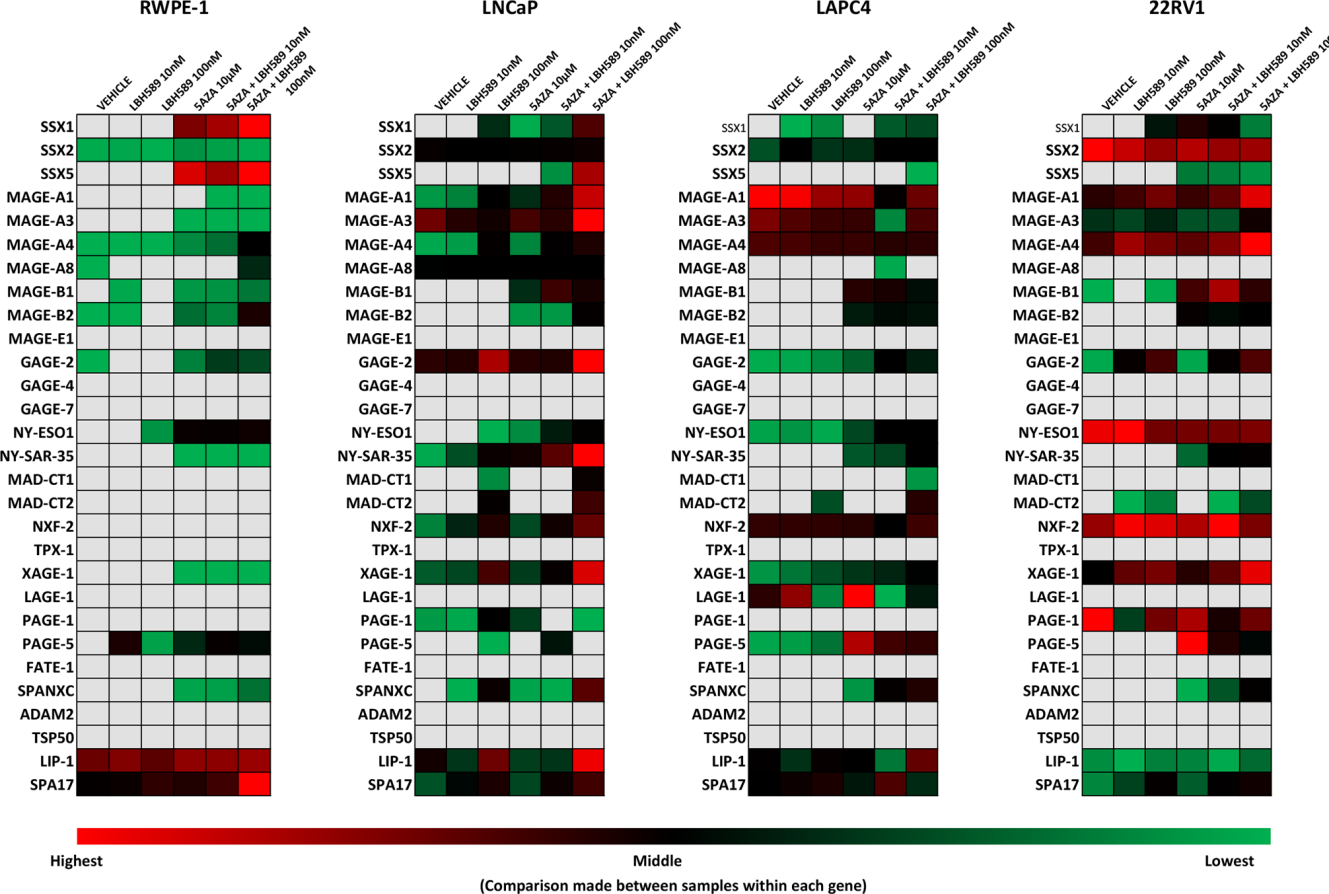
Quantitative analysis of relative expression of CTA mRNA in androgen-receptor expressing PC cell lines and in RWPE-1 normal epithelial cells in response to EMA treatment RNA was evaluated by qRT-PCR for expression relative to an internal control transcript (P0) following EMA treatment of RWPE-1, LNCaP, LAPC4, and 22rv1 cells. qRT-PCR was performed using primers specific for each gene, conducted in triplicate, and repeated in an independent experiment. Expression relative to P0 was color scaled from red (highest) to black (middle; 50th percentile) to green (lowest) for each CTA separately, across all cell lines, thus similar colors between different rows cannot be directly compared. Grey indicates that no relevant expression was detected.
